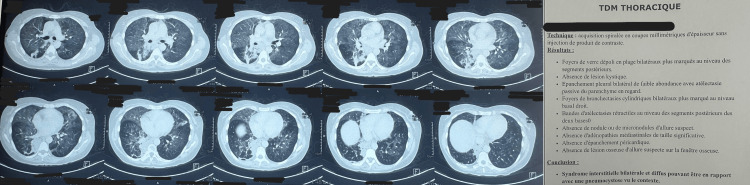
CT images showing diffuse bilateral ground‑glass opacities (left panel) and the corresponding radiology report (right panel).
